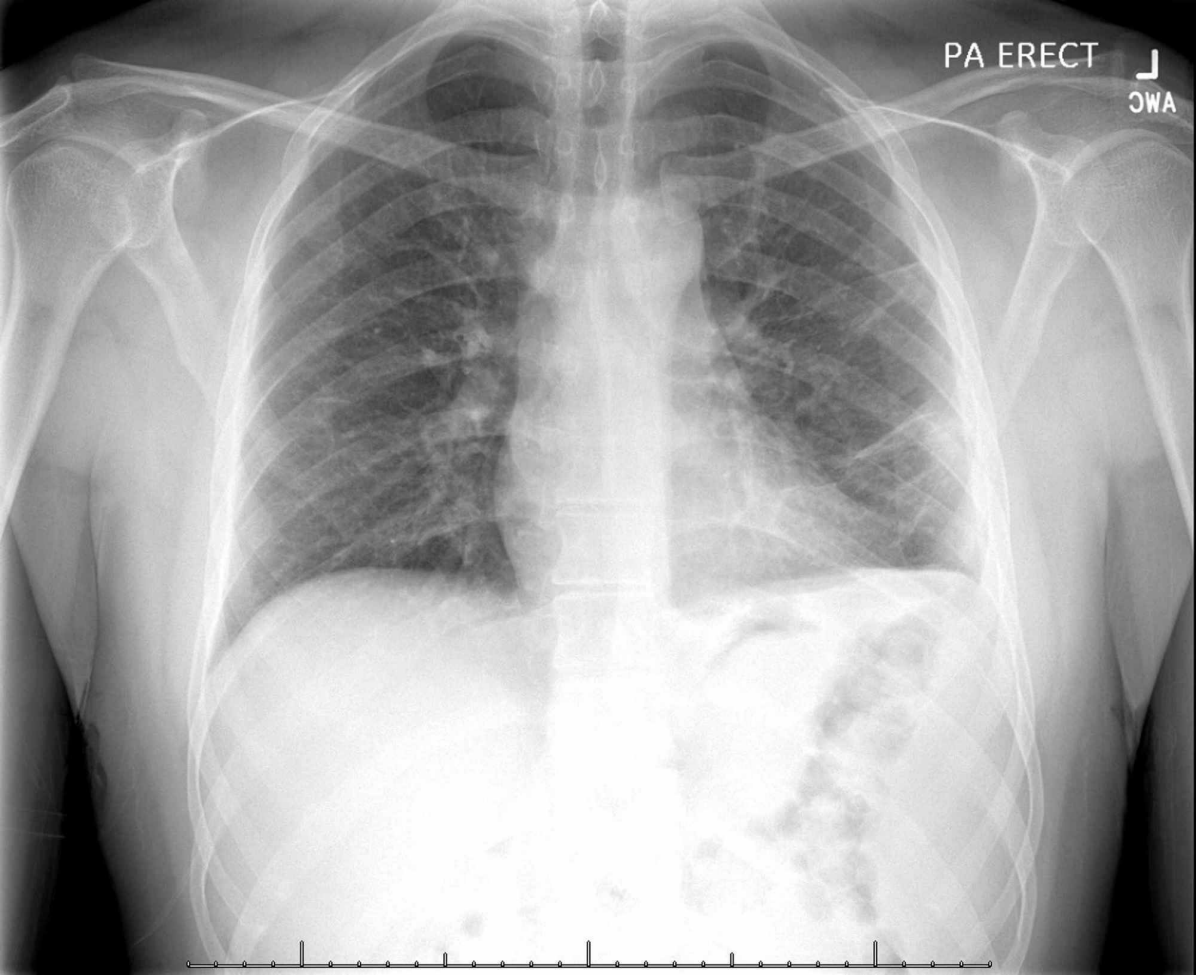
Follow-up chest x-ray, months into anti-tuberculous therapy showing significant improvement.
